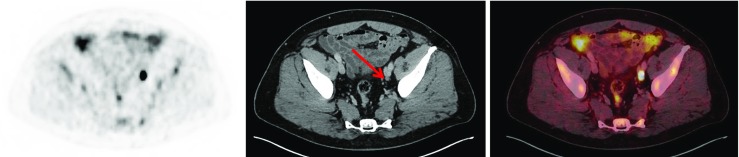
^18^F-PSMA-1007 PET/CT imaging (axial images: *left* PET, *centre* CT, *right* fused PET/CT) in a 59-year-old patient with biochemical relapse (PSA level 0.54 ng/ml) after radical prostatectomy and with a Gleason score of 7b (4 + 3). Images show a 5-mm lymph node lesion (*arrow*) with high tracer uptake along the left iliac artery
